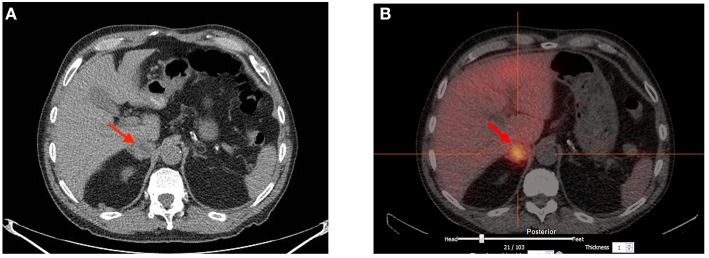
(A) Computed Tomography scan showing a 3 cm heterogeneous right adrenal mass of undetermined significance. (B) Strong Meta-iodobenzylguanidine (MIBG) uptake of the 2 × 2 × 3 cm right adrenal mass with no evidence of distant metastasis.
